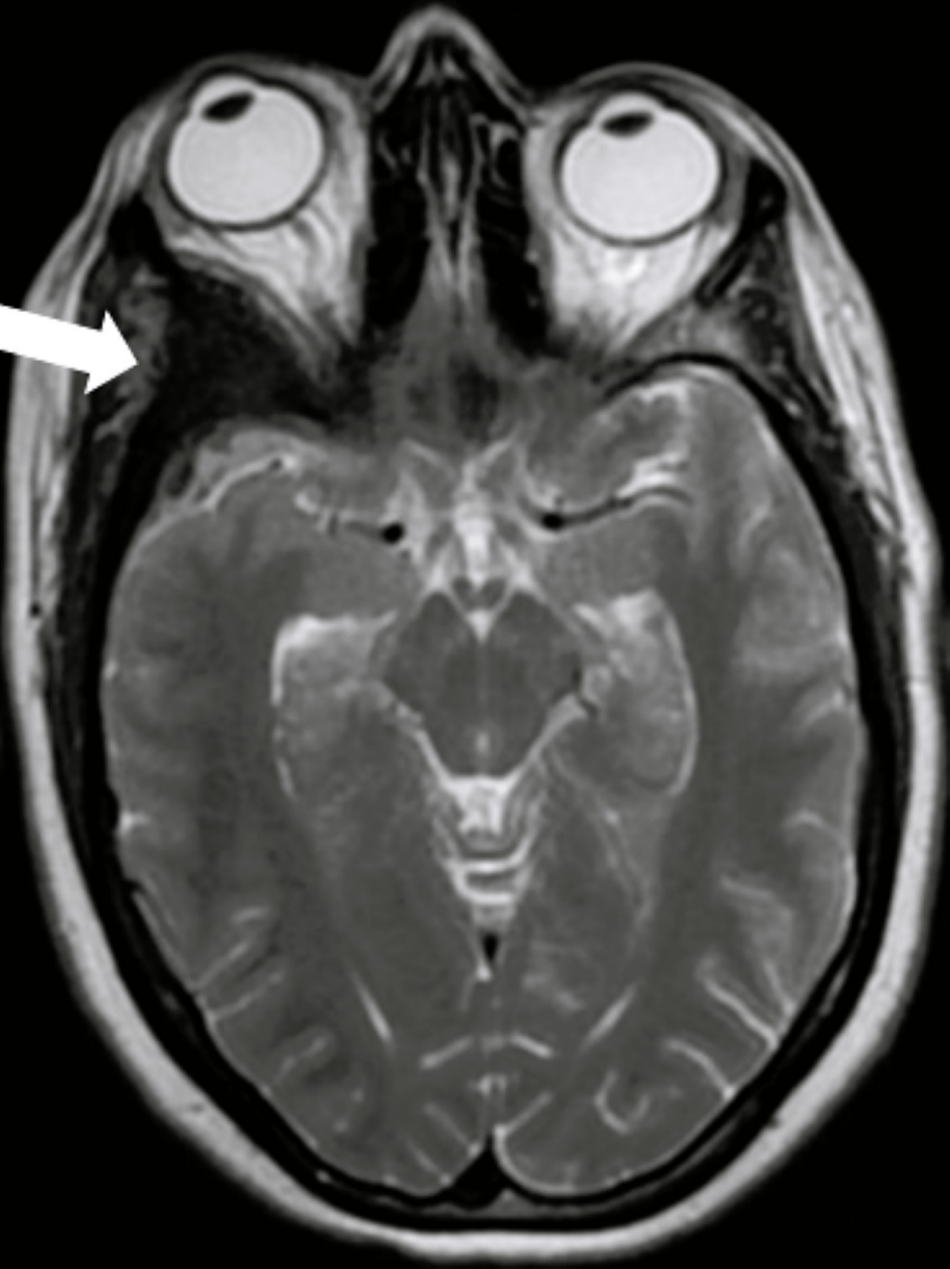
Brain MRI axial sequence Right spheno-fronto-temporo-orbital intraosseous lesion in hyposignal on the T2 sequence (white arrow).
